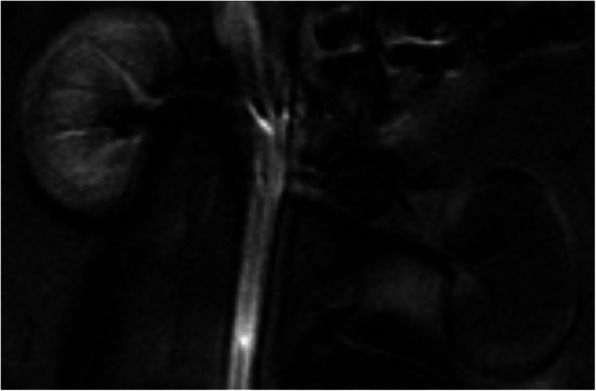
MRI done immediately after embolization (M0) of the left renal artery with 25% Glubran®2. Coronal T1 view after contrast injection at the arterial phase showing occlusion of the left renal artery with no enhancement of the left renal parenchyma
